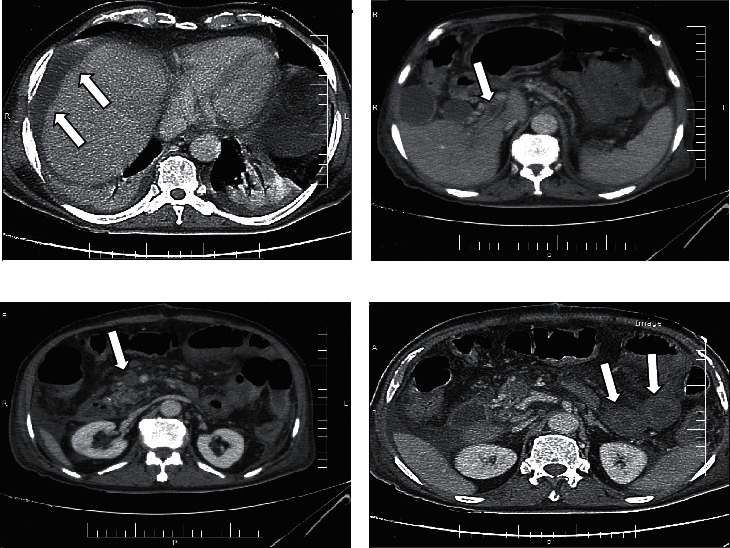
The patient's CT: (a) ascites in chest CT; (b) portal vein thrombosis in abdominal CT; (c) extension of thrombosis in the superior mesenteric vein in abdominal CT; (d) edematous small bowel wall in abdominal CT.
